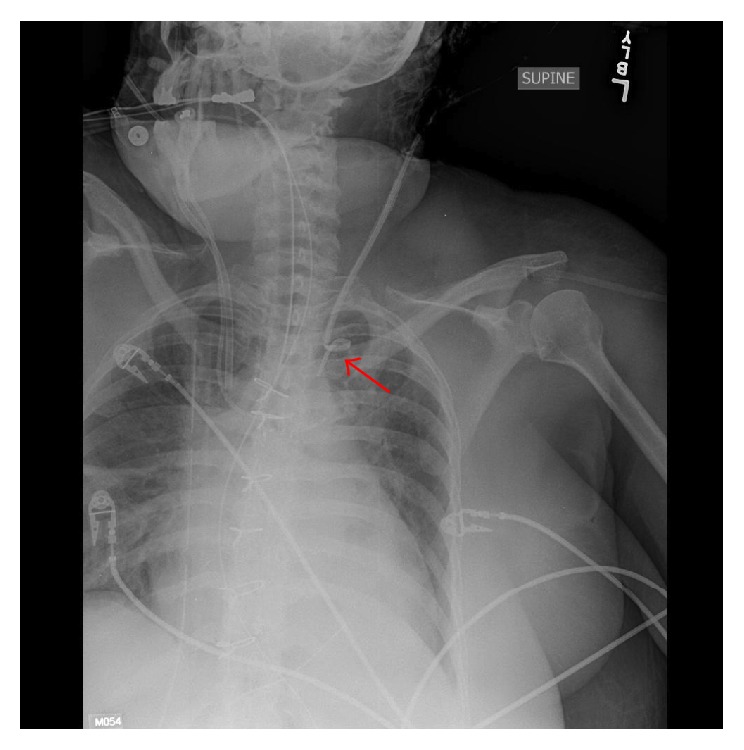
Chest radiography of kinked PAC at the confluence of the left internal jugular vein and left subclavian vein.
